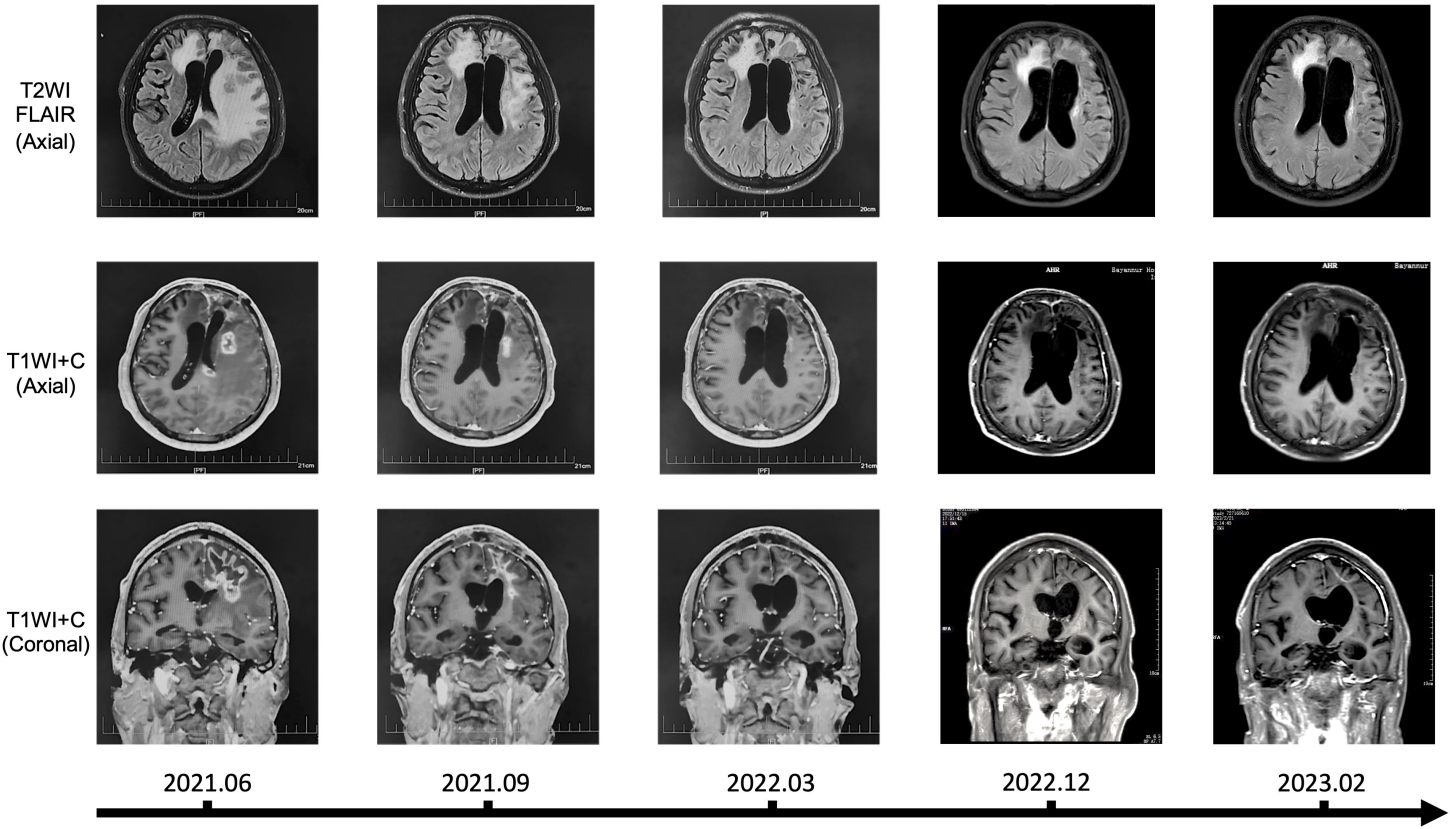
MRI findings over time in a patient treated with CHA.
